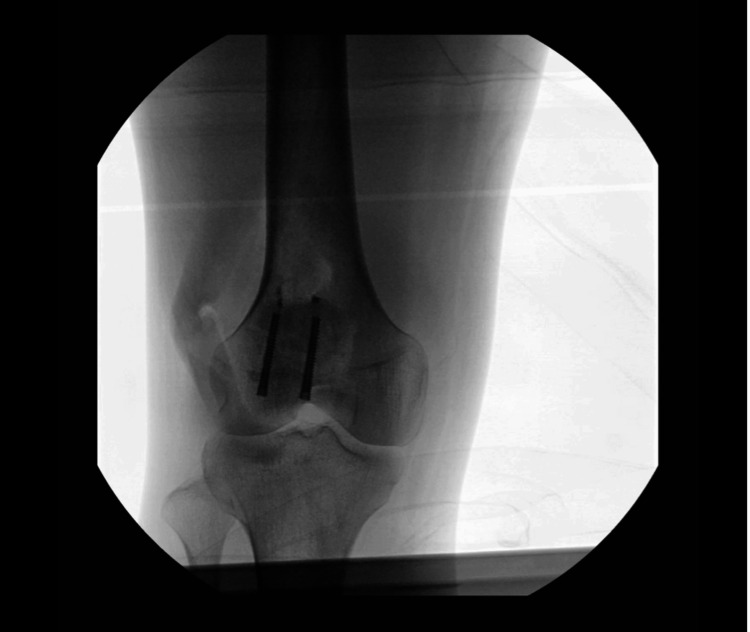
X-ray of the right knee after open reduction and internal fixation.
